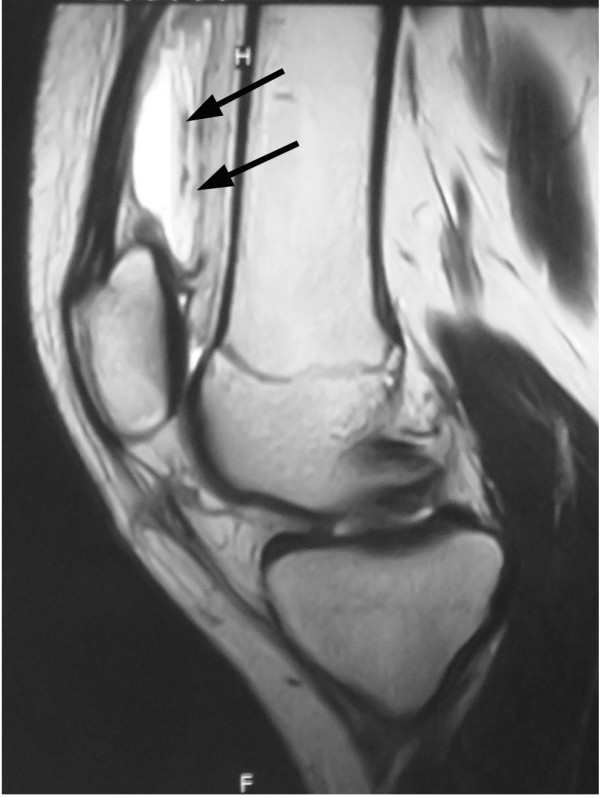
T2 weighted MR image shows high intensity mass in the suprapatellar pouch (arrows).
